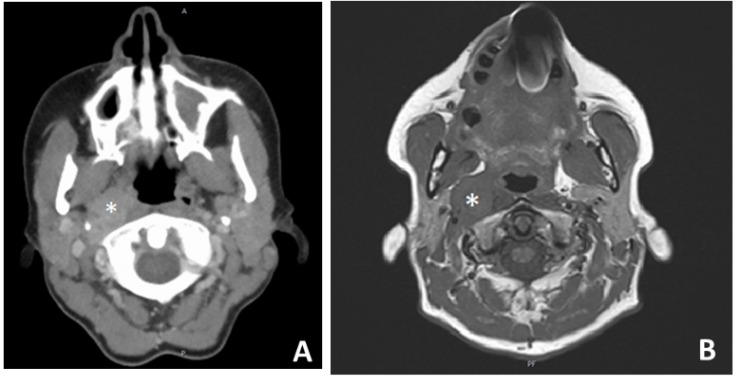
Axial contrast-enhanced computed tomography (A) and T1-weighted MRI (B) of a patient with regional recurrence in the right parapharyngeal space (white asterisk) 68 months after initial craniofacial resection and adjuvant irradiation in olfactory neuroblastoma Kadish–Morita stage B/Hyams grade III.
